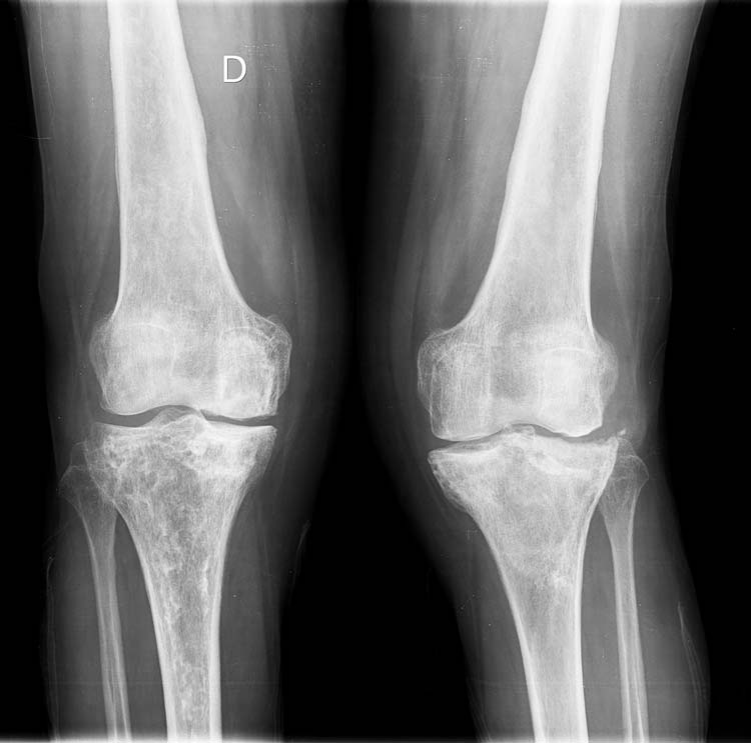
Initial both-legs standing radiographs of the knee. It showed a pattern of diffuse osteopenia with areas of sclerosis, lateral extended bone reaction and decreased lateral articular space (Kellgren–Lawrence 3) in the left knee. Bone necrosis with decreased medial articular space (Kellgren–Lawrence 3) were observed in the right knee.
